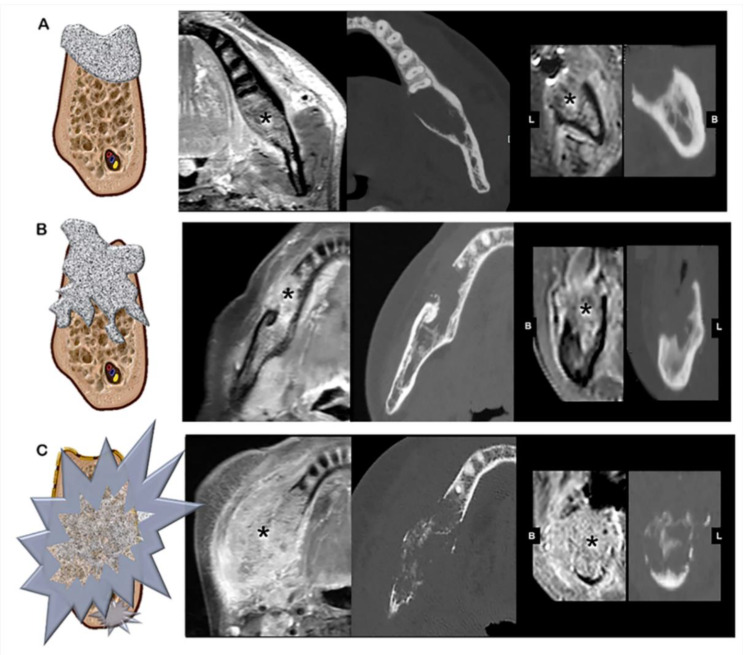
Imaging type classification. (A) Type I, smooth and round, with perilesional bone resorption, (B) Type II, rough and irregular bone margin around the lesion, and (C) Type III, extensive and unpredictable infiltration of the lesion into the buccal or/and lingual cortical bone. Panels on the right indicate a tumor mass on magnetic resonance imaging (MRI) and computed tomography (CT). Image sequences are, from left to right, contrast-enhanced T1-weighted MRI axial view, CT axial view, contrast-enhanced T1-weighted MRI coronal view, and CT coronal view.
